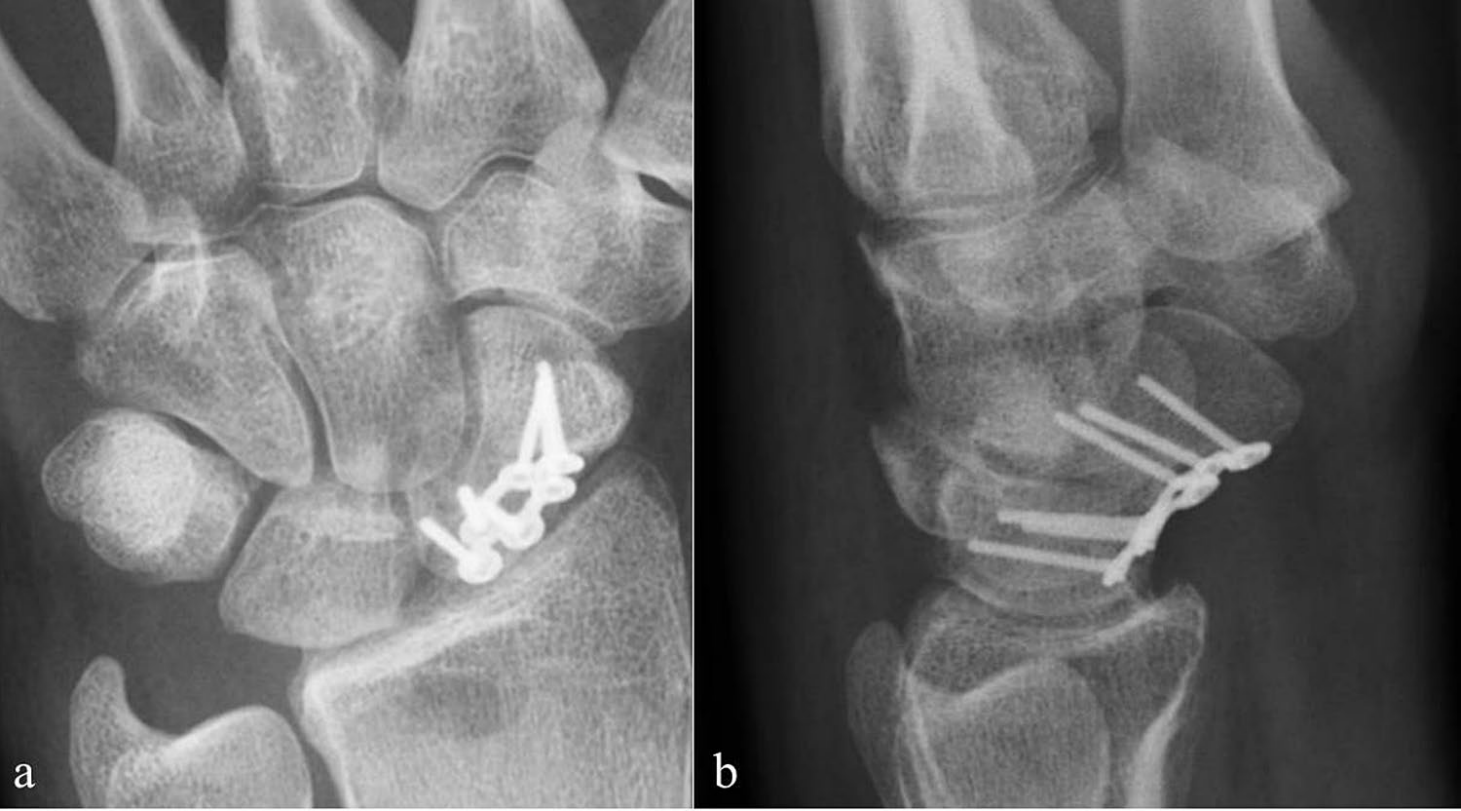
Control X-ray anteroposterior (a) and lateral (b) with the consolidation of the right scaphoid 6 months postoperative.
